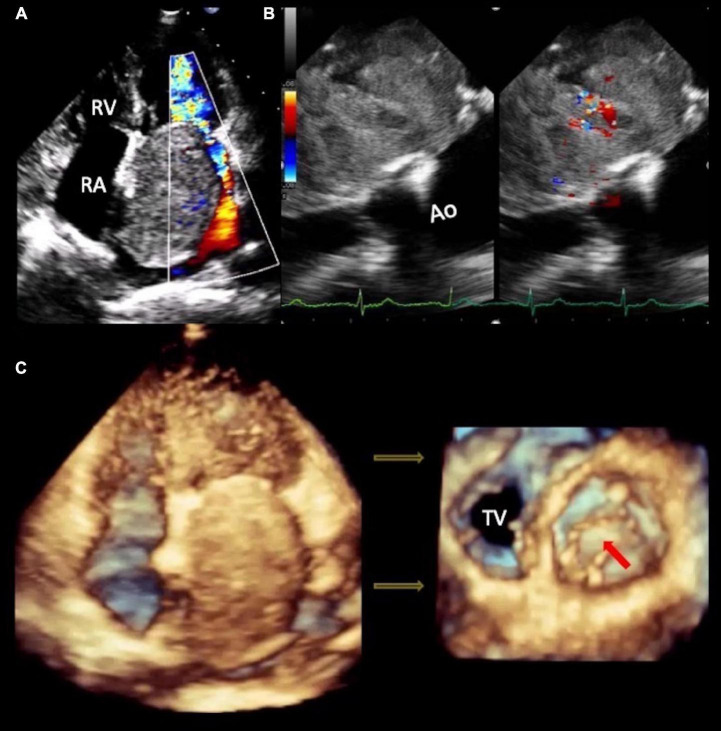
Echocardiogram. (A) 2D-TTE four-chamber view shows a large echogenic oval mass that occupies practically the entire left atrium, with regular borders, heterogeneous echogenicity, adhered to the interatrial septum by a short pedicle and moderate to severe mitral regurgitation in the color Doppler. (B) 2D-Transesophageal echocardiogram and color-Doppler evidenced significant vascularization inside the mass. (C) 3D-TTE shows the mass protruding through the mitral valve into the left ventricle during end-diastole (Supplementary Video 2). Ao, aorta; RA, right atrium; RV, right ventricle; TTE, transthoracic echocardiogram; TV, tricuspid valve.
